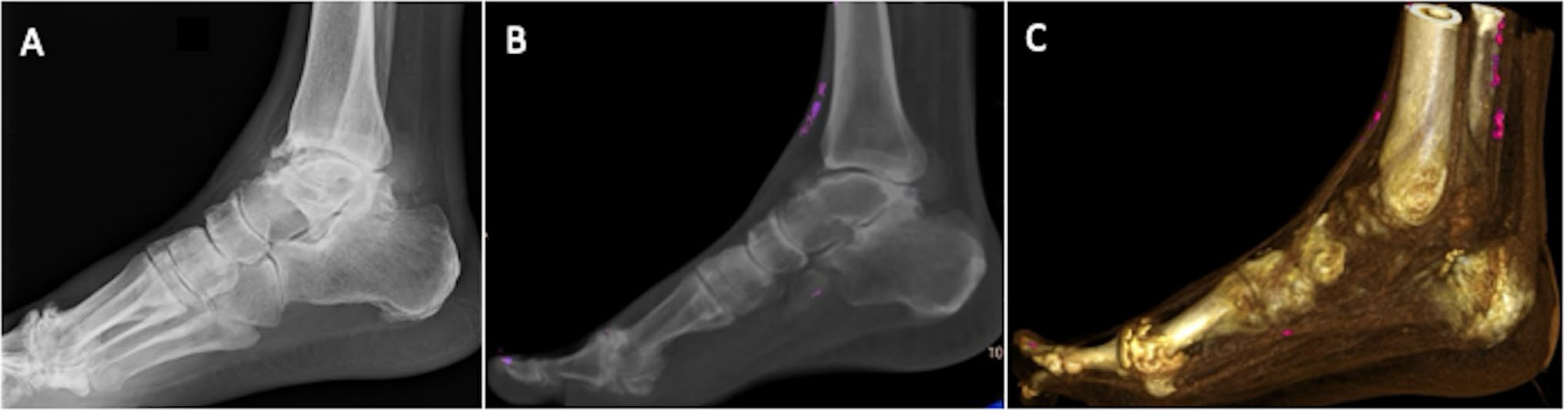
Acute ankle pain in 72-year-old male patient with gout. Lateral X-ray view a shows osteoarthritis of the tibio-talar and first metatarsophalangeal joints. Post‐processed sagittal average intensity projection (b) and volume-rendered (c) color-coded DECT images show urate deposits colored in purple
